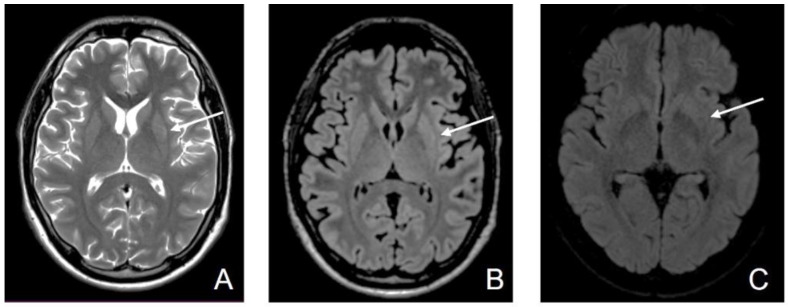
Fiveth MRI (D63). (A) Axial T2-wi; (B) Axial FLAIR: normal signal intensity with only a discrete band of peripheral high signal intensity T2 wi/FLAIR in bilateral putamen; (C) Axial DWI: there is no more restricted diffusion in the lenticular nucleus (white arrow).
